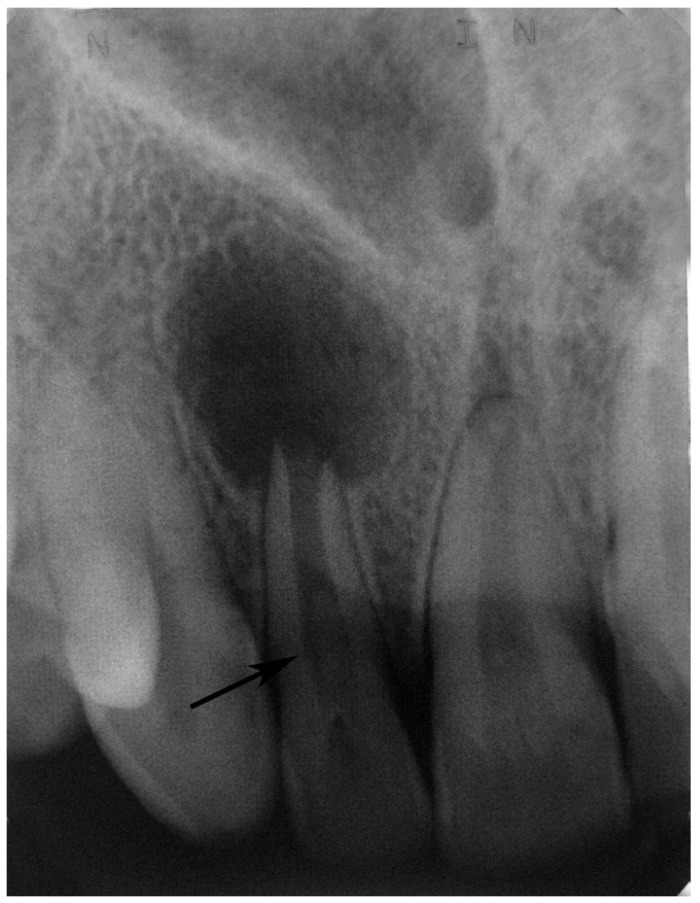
Maxillary right lateral incisor with periapical lesion affected by Type III dens invaginatus (arrow).
